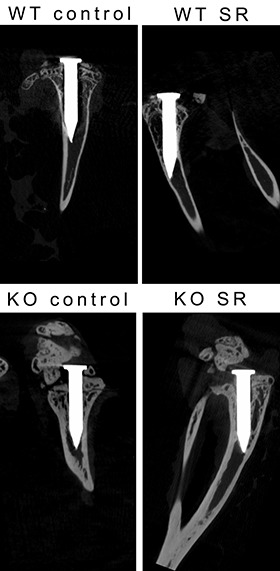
Sagittal-sectional micro-computed tomography scans of titanium implants. SR: strontium ranelate; WT: wild type; KO: knockout.
